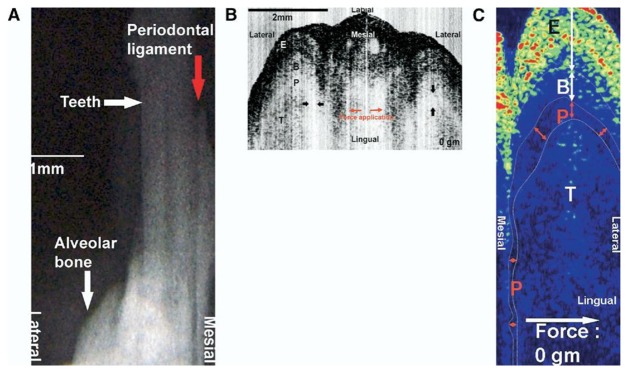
Images of a periodontal ligament. (A) Radiograph. (B) OCT. (C) Logging OCT images; the boundary of each tissue can be identified more clearly (reprinted from reference [82]).
